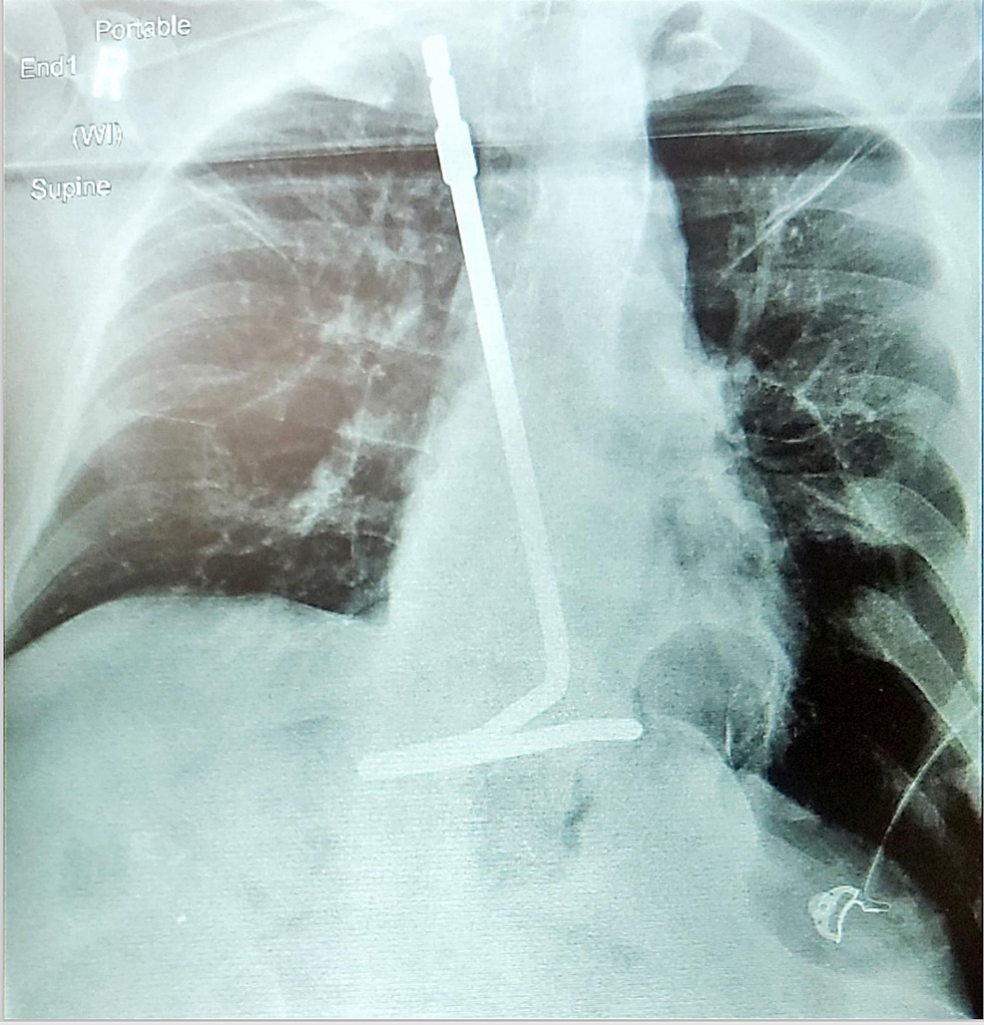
Left-sided pneumothorax on chest X-ray
